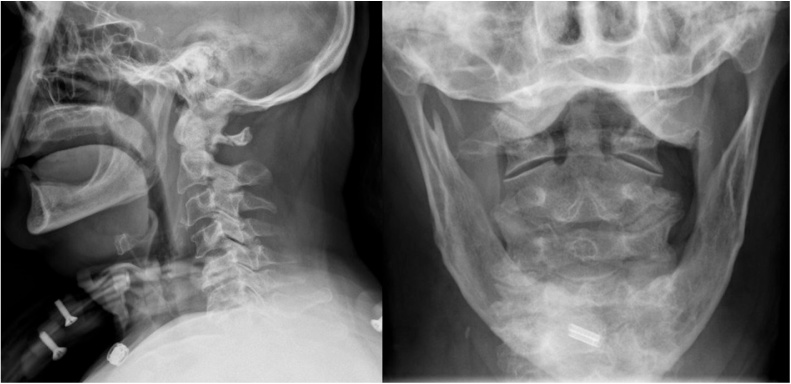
Post reduction X-rays of the cervical spine (lateral and open mouth views).
